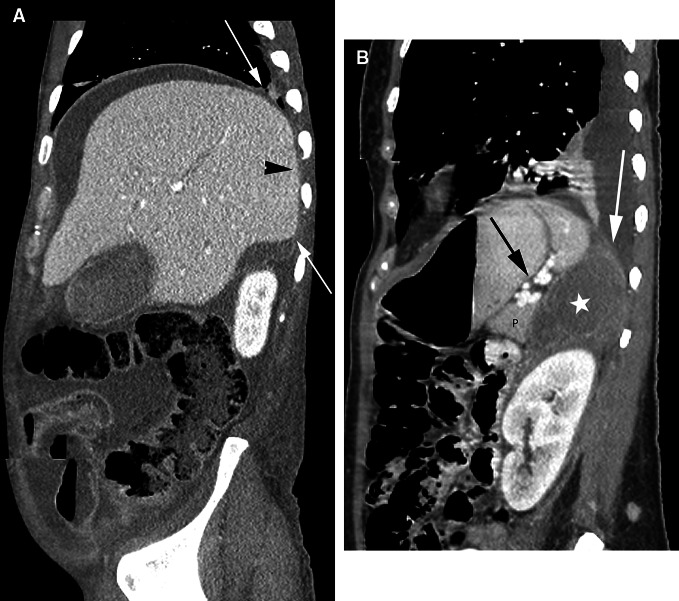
Extraperitoneal spaces in the upper abdomen. A Sagittal CT image shows the peritoneal reflections over the liver (*arrows*). The liver posterior and medial to these reflections is left bare of peritoneum and abuts the diaphragm (*arrowhead*). Note that peritoneal fluid surrounds the liver except for the bare area. B Sagittal CT image shows fluid in the left perinephric space (*asterisk*) extending superiorly to abut the diaphragm (*white arrow*). The fluid is posterior to the pancreas (P) and the splenorenal ligament (*black arrow*).
